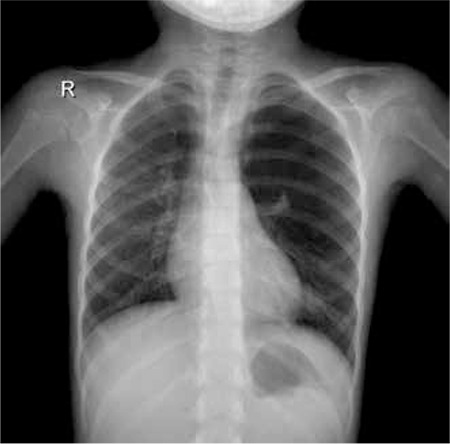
Posterior anterior lung radiography imaging of the patient.
